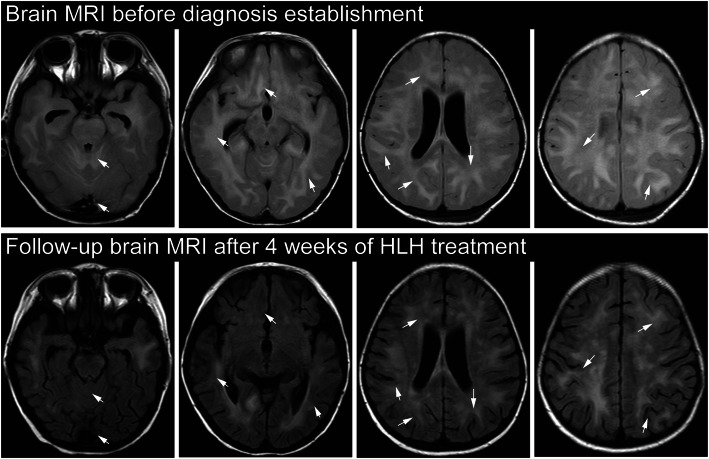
Brain MRI presentation of neurological abnormalities in fluid-attenuated inversion recovery (FLAIR) images. Brain MRI before diagnosis establishment (top row) showed extensive hyperintense lesions involving the bilateral cerebrum, cerebellum, brainstem, basal ganglia, thalamus and corpus callosum, with nodular enhancement (arrow). Follow-up brain MRI after 4 weeks of HLH treatment (bottom row) showed that most of the lesions were attenuated (arrow), and especially the original hyperintense lesions in cerebellar hemisphere and genu of corpus callosum disappeared
